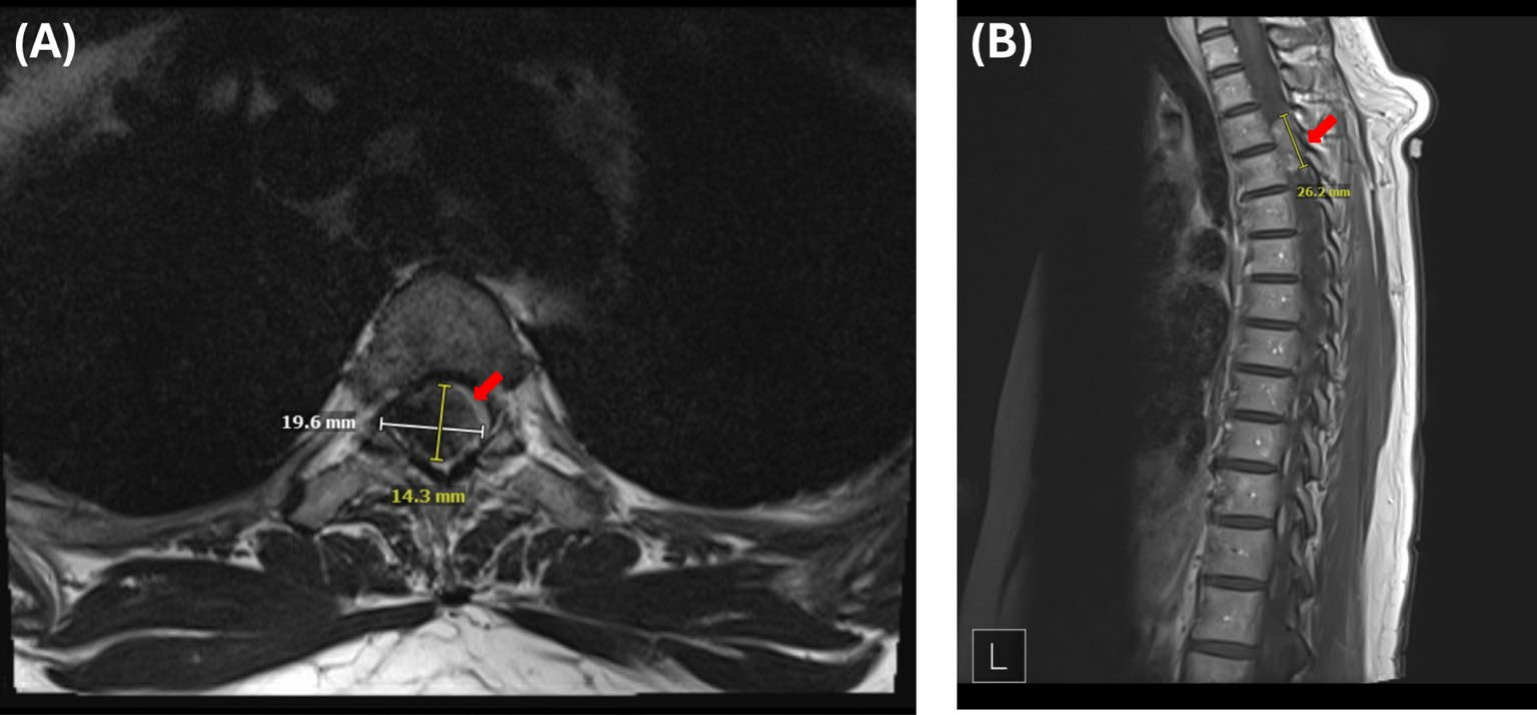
Pre‐operative MRI of the thoracic spine with and without contrast, showing an approximately 2.6 × 2.0 × 1.4 cm calcified mass at the T3–T4 level, consistent with a benign meningioma (Red Arrows). Images are presented in (A) axial and (B) sagittal planes.
